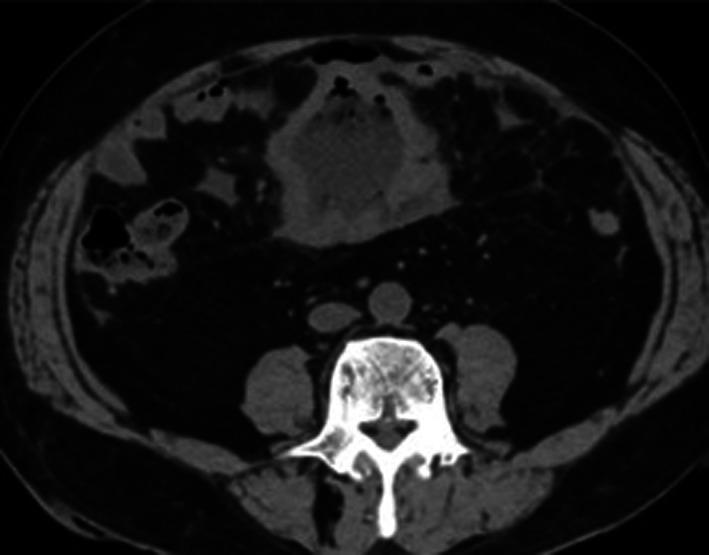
Abdominal computed tomography (CT) scan image before surgery. Abdominal CT scan revealed an 82 × 79 mm thick-wall mass connected to the small intestine and also intra-abdominal free air
